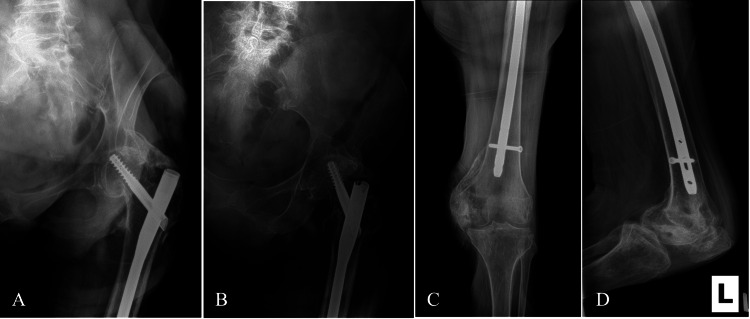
Six-week postoperative radiographs of the left femur A) Anteroposterior view of the left proximal femur demonstrating mild collapse of the fracture and 1 mm of screw cut-out. B) Lateral view of the left proximal femur. C) Anteroposterior view of the left distal femur. D) Lateral view of the left distal femur.
